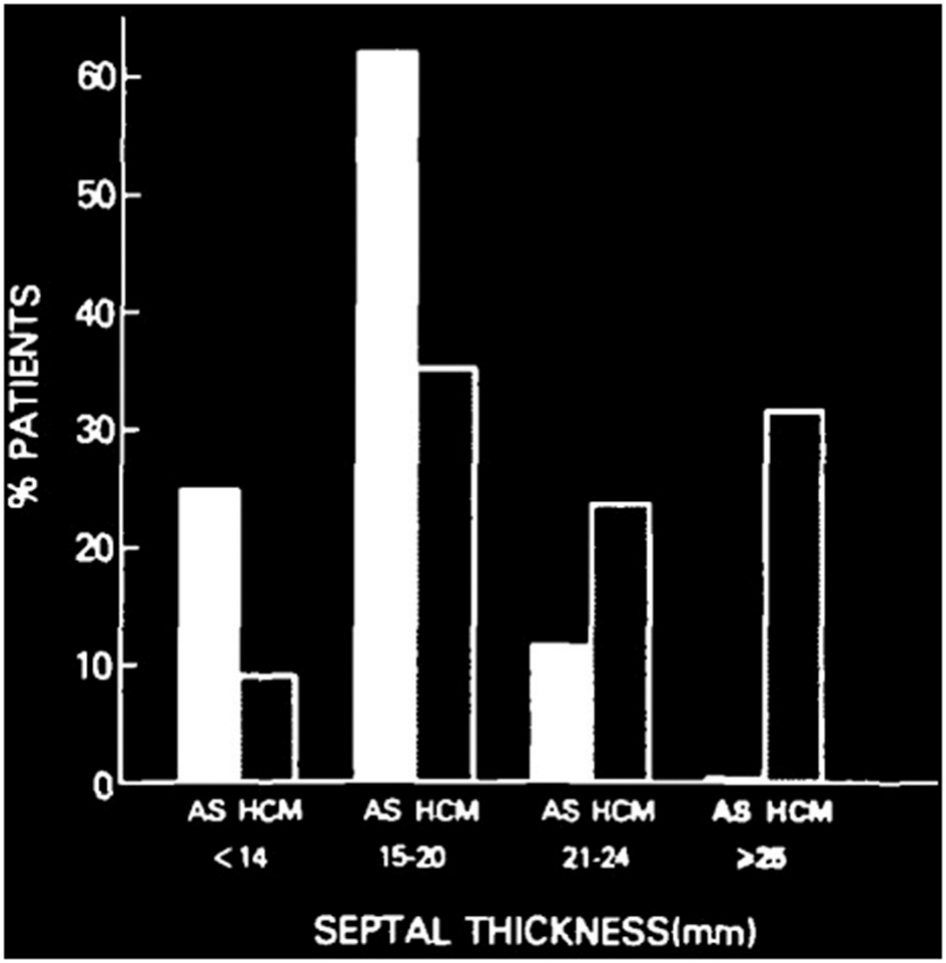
Difference of ventricular septal thickness between valvular aortic stenosis and hypertrophic cardiomyopathy. Comparison of ventricular septal thickness in 85 patients with valvular aortic stenosis (AS) and 151 patients with hypertrophic cardiomyopathy (HCM), studied with echocardiography and at necropsy (5).
